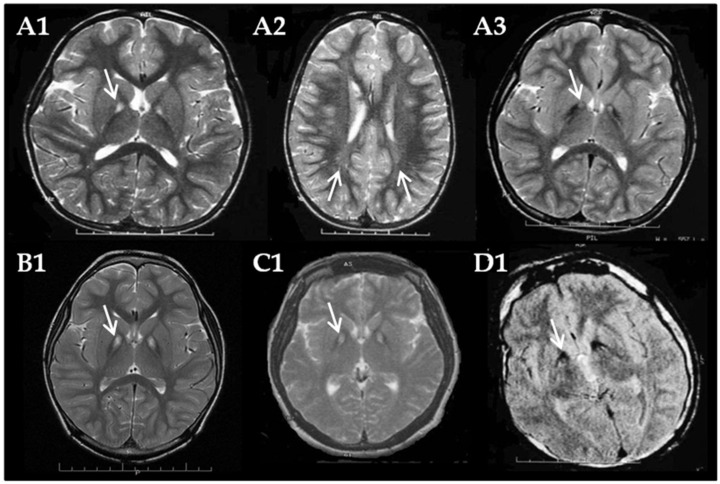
Full MRI progression of globus pallidus involvement in four patients with PKAN (A1–D1). (A1–A3) Imaging of case 1. At age of 3 years, bilateral hyperintensity of the globus pallidus (white arrow) (A1) and abnormal periventricular white matter signal (white arrows) (A2) are observed. At age 9, the characteristic “eye-of-the-tiger” sign is evident (white arrow) (A3). (B1) Case 2 showing the “eye-of-the-tiger” sign (white arrow). (C1) Case 3 with the “eye-of-the-tiger” sign (white arrow). (D1) Case 4 showing bilateral hypointensity of the globus pallidus (white arrow).
